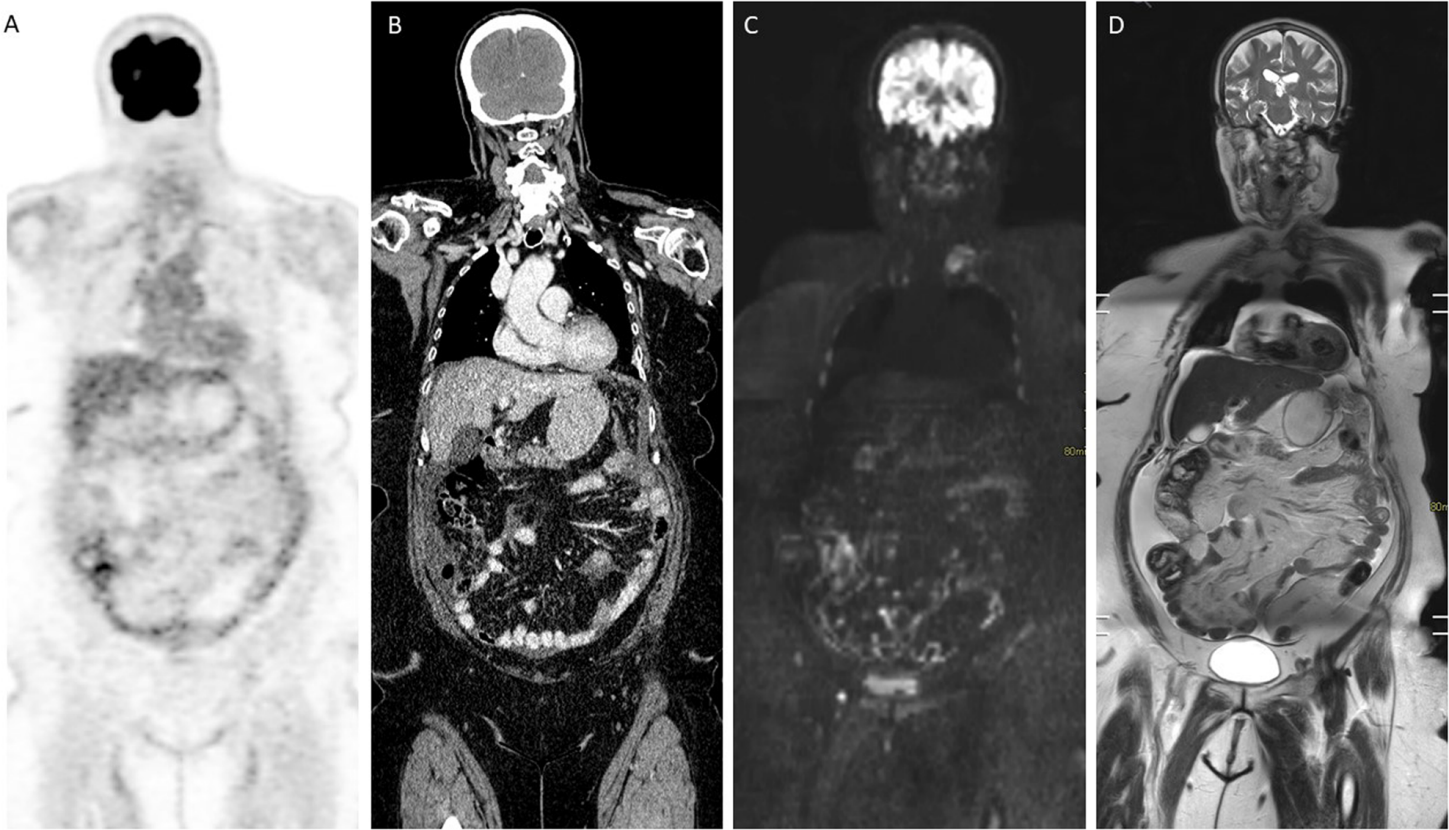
Patient presenting with a new diagnosis of fallopian tube cancer. A, B FDG-PET/CT imaging reveals faint diffuse uptake over the small bowel mesentery, accompanied by non-specific minimal mesenteric infiltration and ascites seen on CT. C, D Additional WB-DWI/MRI shows diffuse linear and micronodular infiltration of the small bowel mesentery and serosa, along with the presence of ascites on coronal reformatted DWI and T2-weighted sequences. Laparoscopy confirmed extensive presence of diffuse miliary metastases, with an average lesion size of 3 mm
